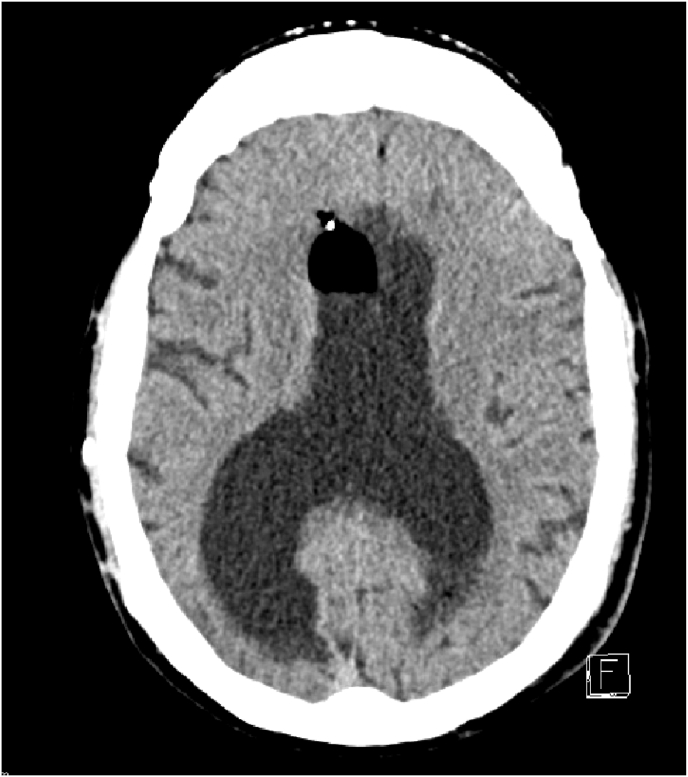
CT SCAN: hydrocephalus with pneumocephalus and pneumoventricle.
